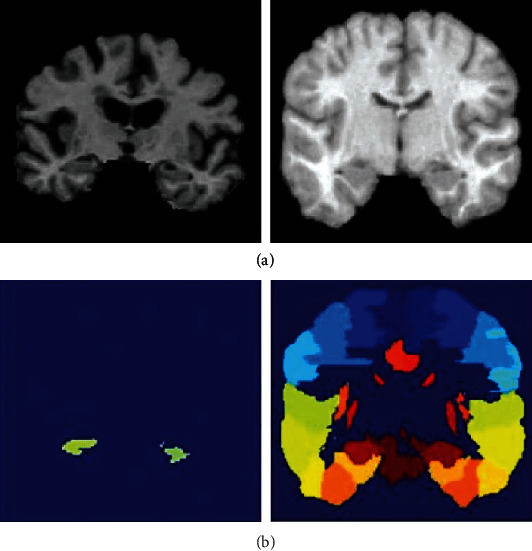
The ADNI dataset MR images: (a) depicts the strength images, and (b) shows the manually segmented labeling.
